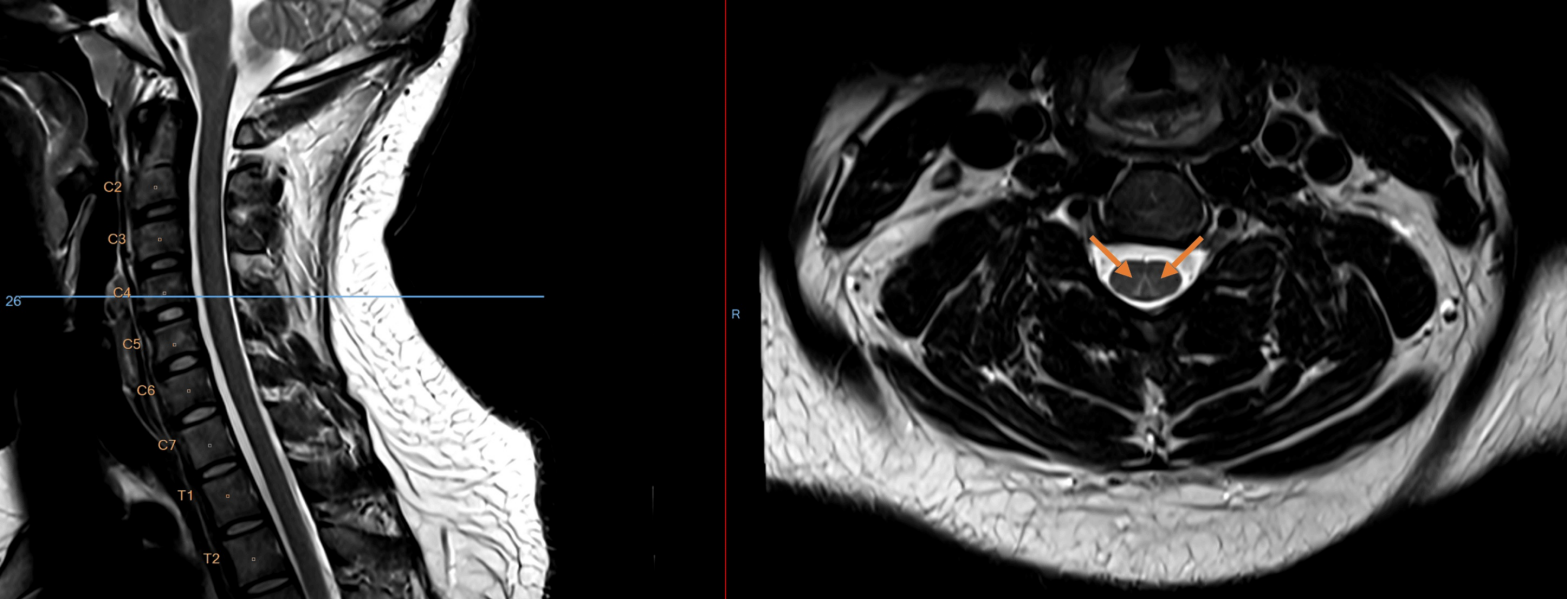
Axial T2-weighted MRI (3T) imaging of the cervical spine (C4)
